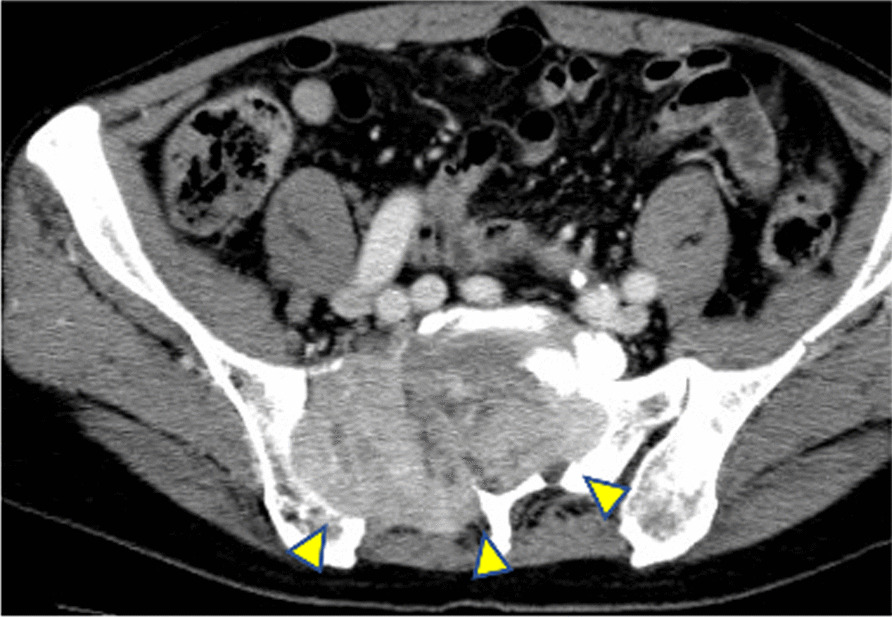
CT image showing 90-mm bone metastasis (indicated by arrows) causing spinal cord compression
